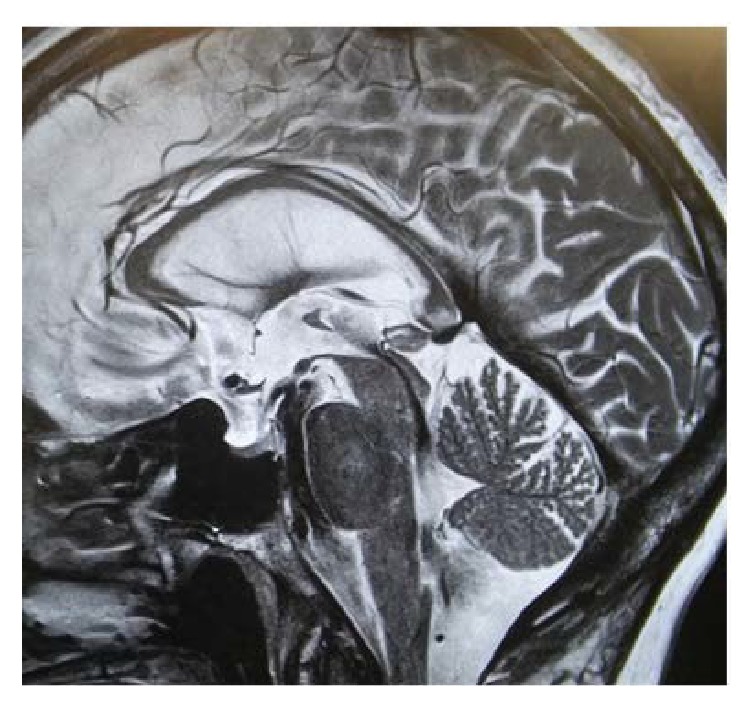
One-year follow-up brain MRI, sagittal T2 section. A strong flow void artifact is noted at the Sylvian aqueduct.
